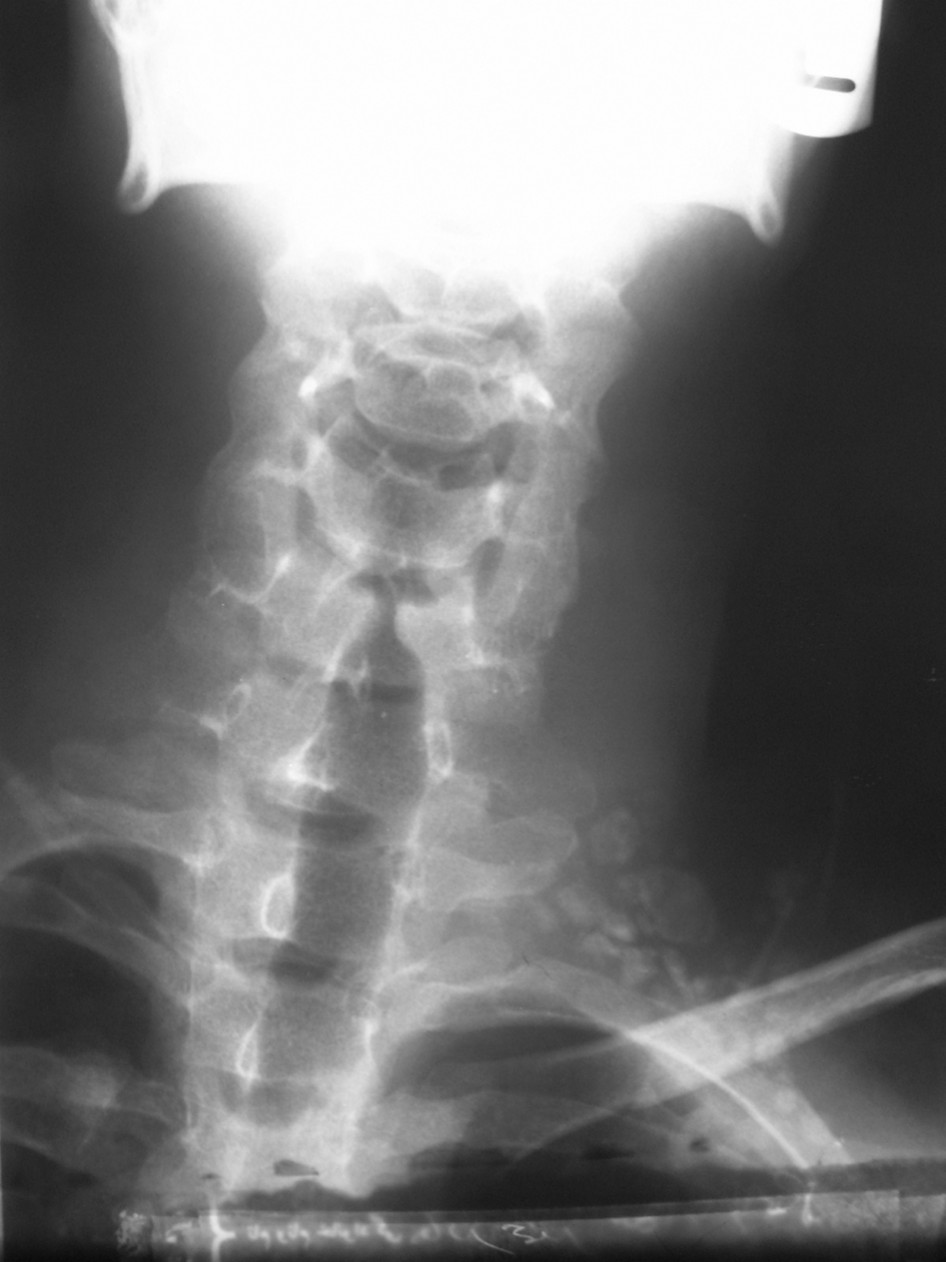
Neck and shoulder X-ray demonstrates geographic and well-circumscribed opacities.
